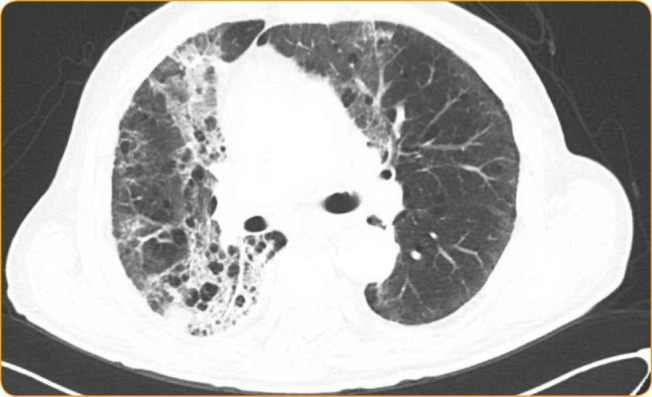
CT scan shows a large part of the radiated right lung and the mediastinum radiation.
